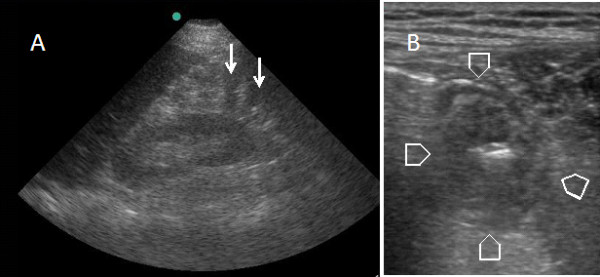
Surgeon-performed bedside ultrasound showing (A) an edematous omentum with hypoechoic stranding in the right upper quadrant of the abdomen (arrows) and (B) a thickened non compressible small bowel in the right lower quadrant of the abdomen (arrow heads).
